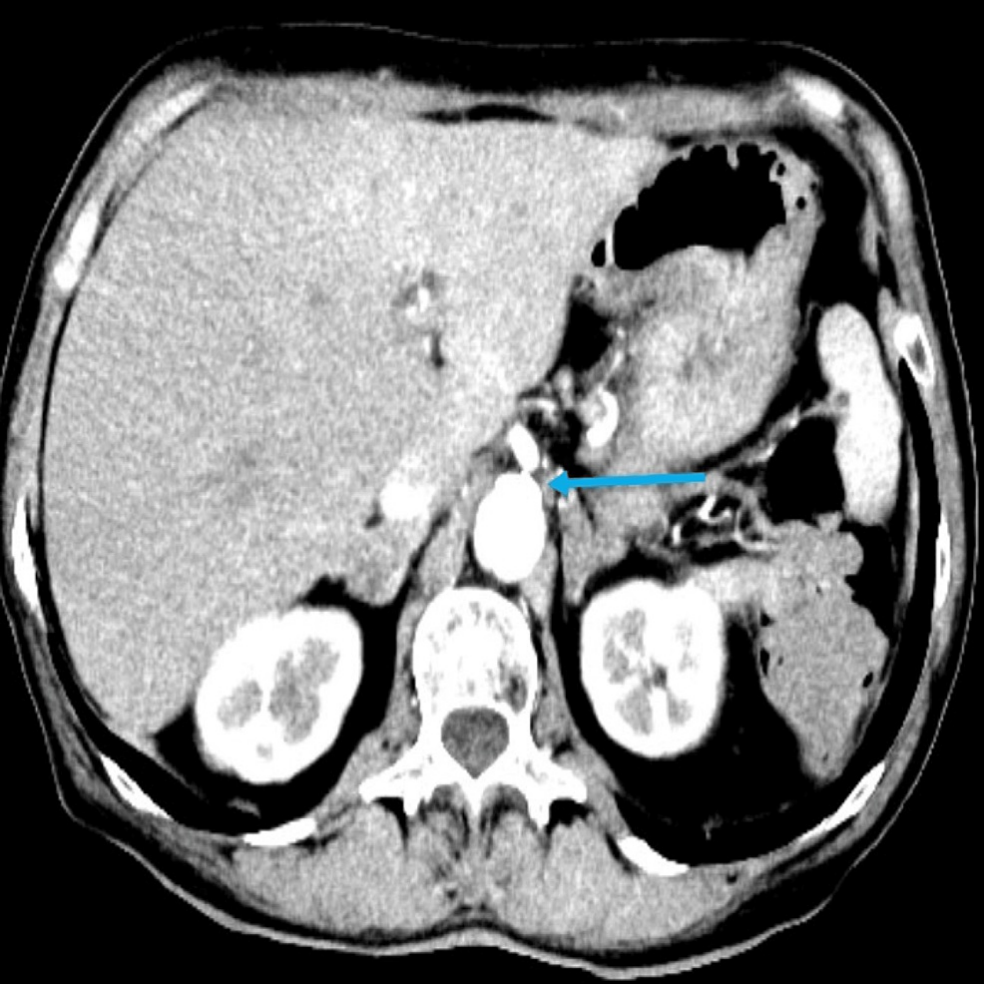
CT angiogram of the abdomen demonstrates a heavily calcified celiac trunk leading to critical stenosis.
